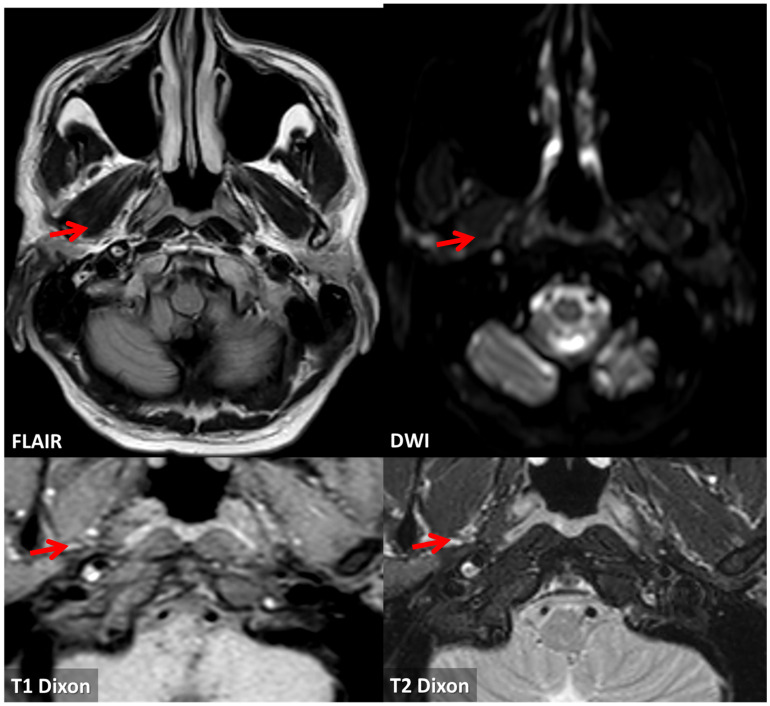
Brain MRI. On FLAIR axial slice, a hyperintense ovoidal structure is evident within the posterolateral wall of the right ICA in the prepetrous segment and a corresponding hyperintense signal is found on DWI (red arrows). The same structure is hyperintense on both T1 and T2 Dixon sequences and the signal features support the diagnostic hypothesis of complicated atheroma with intraplaque hemorrhage and positive remodeling of the corresponding segment.
